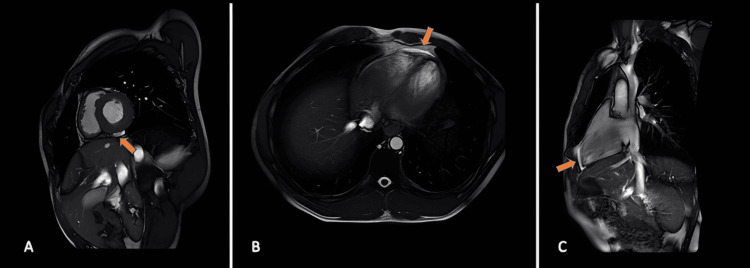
Cardiac MRI Different slices demonstrate hyperintensity of the left ventricular pericardium on T2-weighted sequences. (A) Short-axis view: The left ventricle is seen in cross-section, showing hyperintensity of the left ventricular pericardium on T2-weighted sequences (orange arrow). (B) Four-chamber view: The heart is visualized in a transverse plane, with both left and right ventricles visible. There is a 2 mm thickening of the pericardium with post-contrast enhancement on the T2 sequence (orange arrow). (C) Two-chamber view: The left ventricle and left atrium are seen along their full lengths, showing hyperintensity of the left ventricular pericardium (orange arrow).
